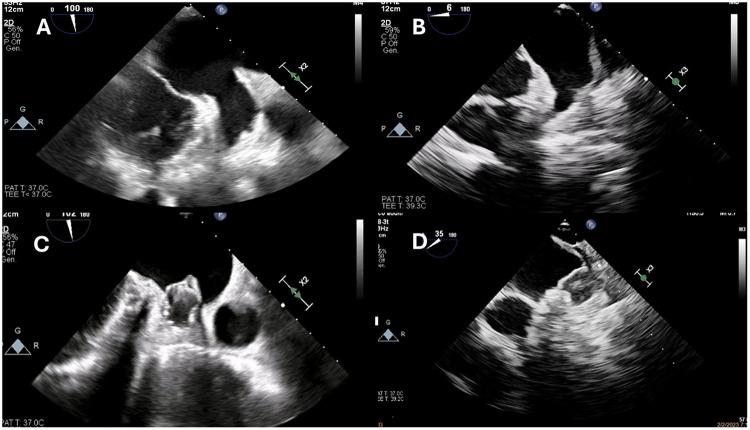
Comparison of imaging quality of standard TEE probe (A,C) and micro-TEE probe (B,D). (A) LAA view with standard TEE probe. (B) LAA view with micro TEE probe. (C) View of closure device in LAA with standard TEE probe. (D) View of closure device in LAA with micro TEE probe. LAA, left atrial appendage; TEE, transoesophageal echocardiography.
